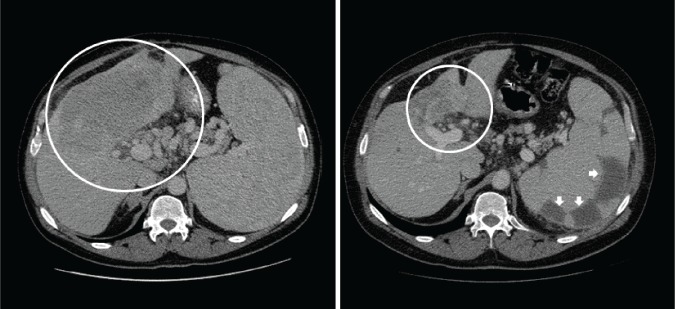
Prolonged partial response for 12.1 months noted in the primary hepatic lesion of a patient with advanced cholangiocarcinoma treated with hepatic arterial infusion of a cytotoxic agent along with intravenous anti-angiogenic agent CT scan of the abdomen showed liver metastases at baseline and after 12 months of treatment. White arrows show multiple areas of hypoattenuation secondary to splenic embolization.
